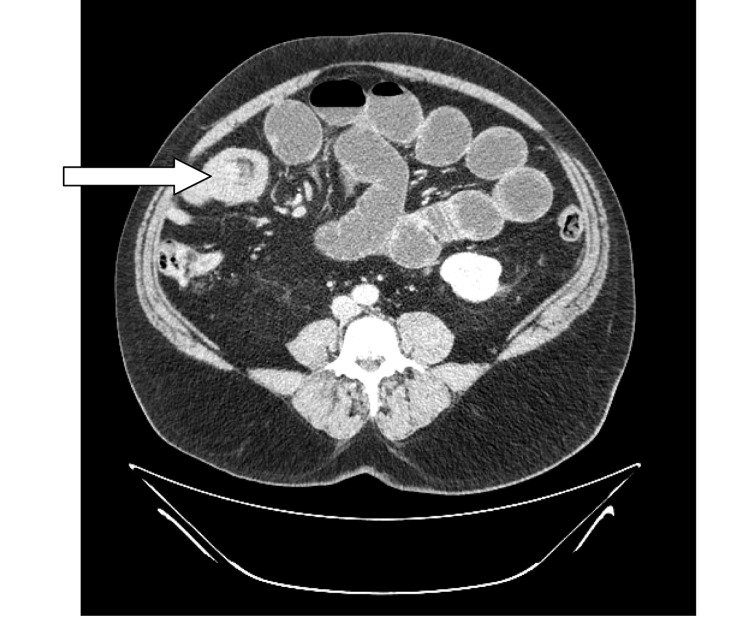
Axial computed tomography of the abdomen with contrast showing small bowel intussusception with ‘target’ lesion (arrow)
